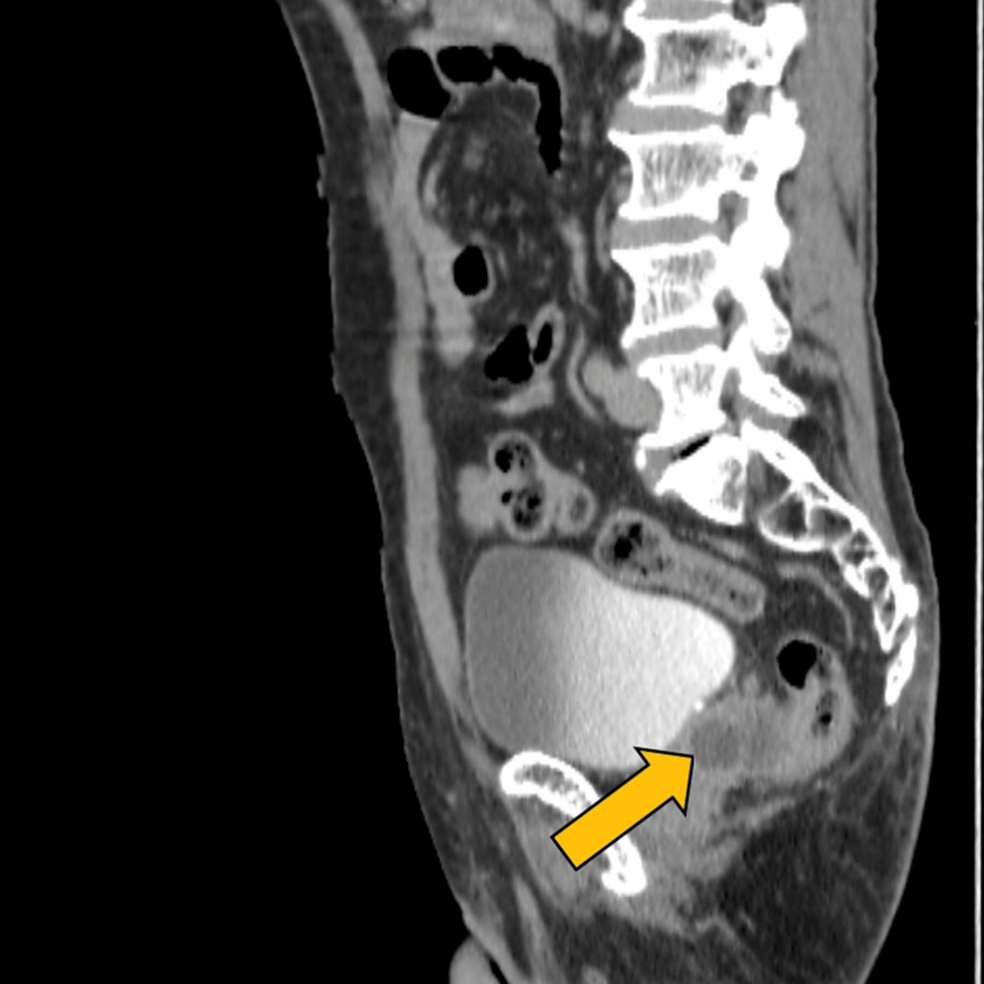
Midsaggital image showing multiloculated hypoechoic lesions with enhancing walls in the prostate CECT: Contrast-enhanced computed tomography.
